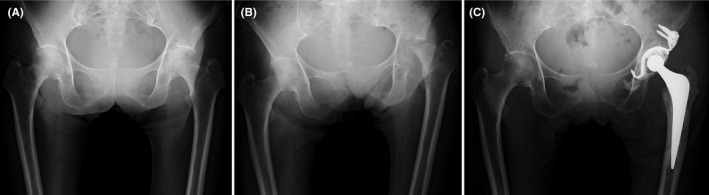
(A) Anteroposterior radiograph of the left hip showing joint space narrowing. (B) Radiograph shows complete destruction of the femoral head. (C) Radiograph shows left total hip replacement
